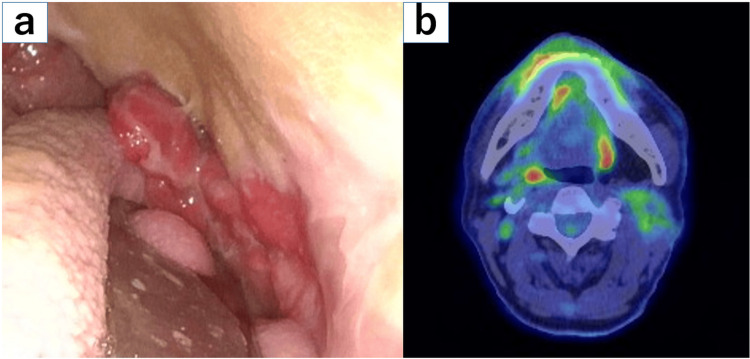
Local recurrence at the inferior margin of the flap after surgery for left buccal mucosa carcinoma. (a) A flexible nasopharyngeal laryngoscopic view showing an irregular elevated lesion at the flap margin. (b) FDG-PET image demonstrating focal uptake corresponding to the recurrent lesion.
